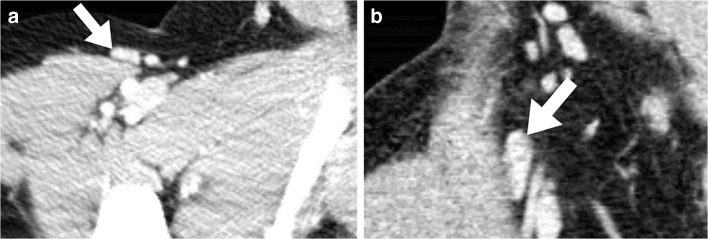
Abdominal contrast-enhanced CT images (120 kV, 50 mAs) in a 15-year-old boy. a Axial reconstruction shows an inguinal lymph node (*arrow*) with a short-axis diameter of 7 mm and a long-axis diameter of 15 mm. b Corresponding image in the coronal plane shows the same inguinal lymph node (*arrow*) with a short-axis diameter of 12 mm and a long-axis diameter of 30 mm
